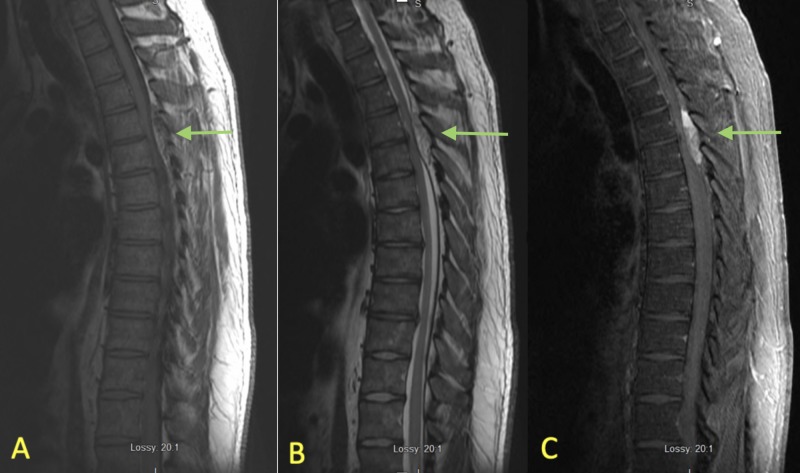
MRI thoracic spine sagittal T1 (A), T2 (B), T1 fat suppression with contrast (C). Panel (A) demonstrates a mixed intensity lesion at the T4-T5 region seen on T1 sequence indicated by the arrow. Panel (B) demonstrates mixed intensity on T2 sequence indicated by the arrow. Panel (C) demonstrates a contrast enhancing lesion indicated by the arrow.
